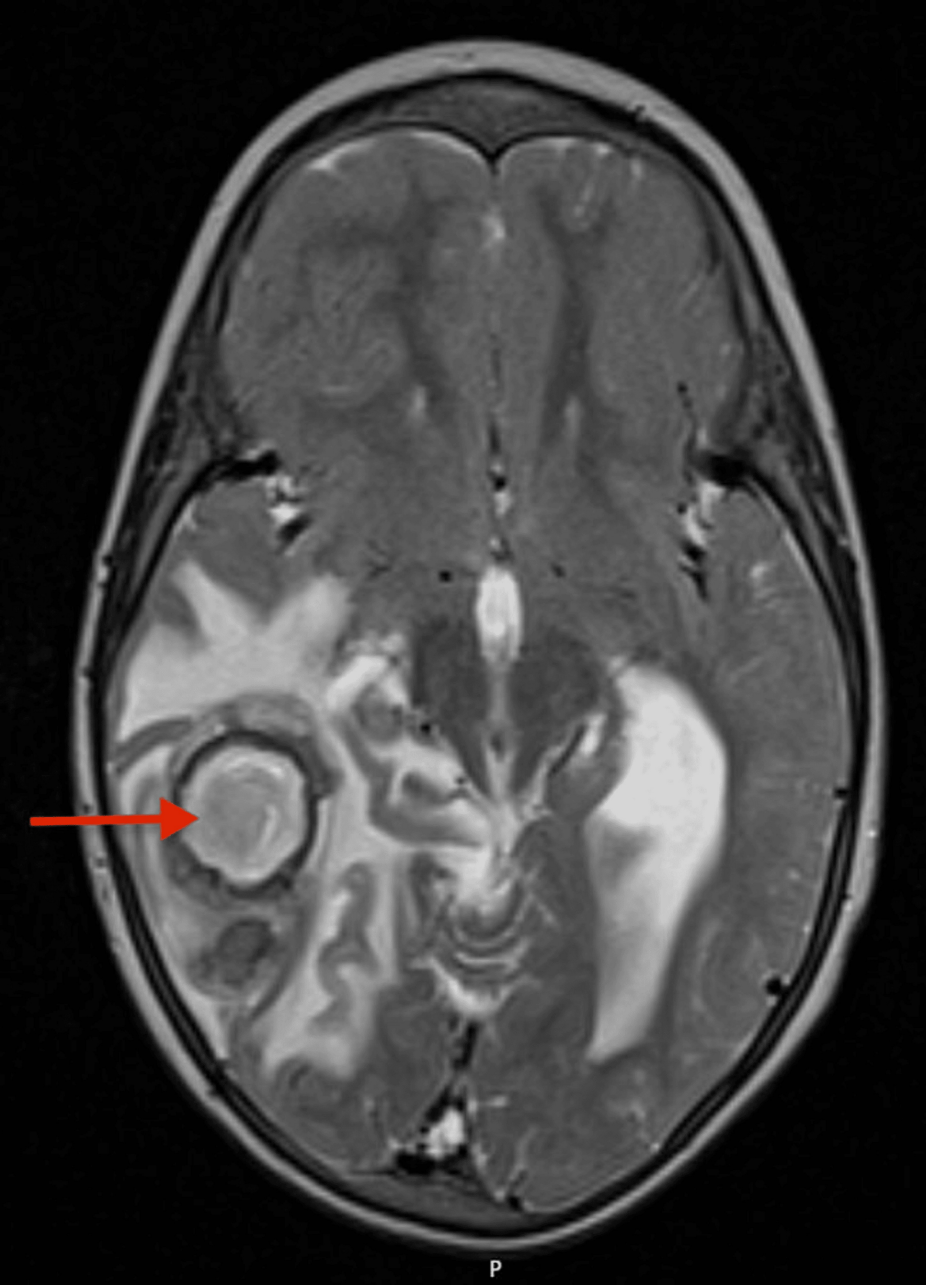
Axial T2-weighted MRI shows a large, well-defined hyperintense right temporal lesion with surrounding vasogenic edema and midline shift
